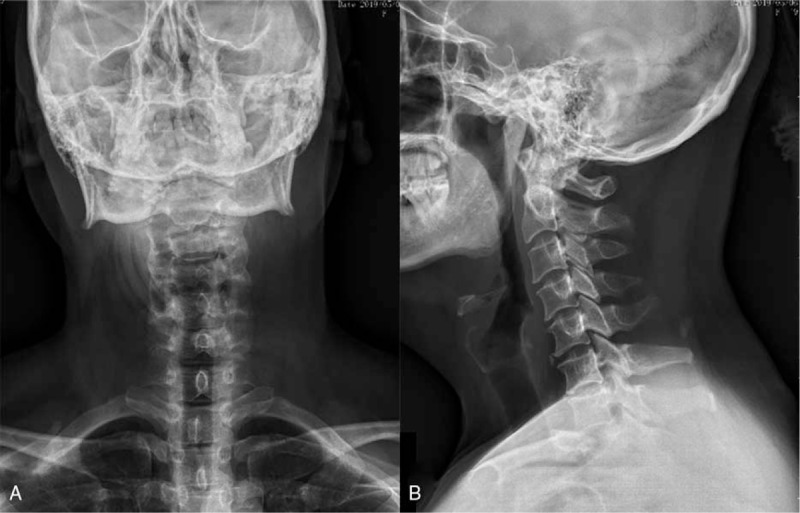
Postero-anterior radiograph (A) and lateral (B) X-ray film of cervical spine showed the cervical curvature became straight.
